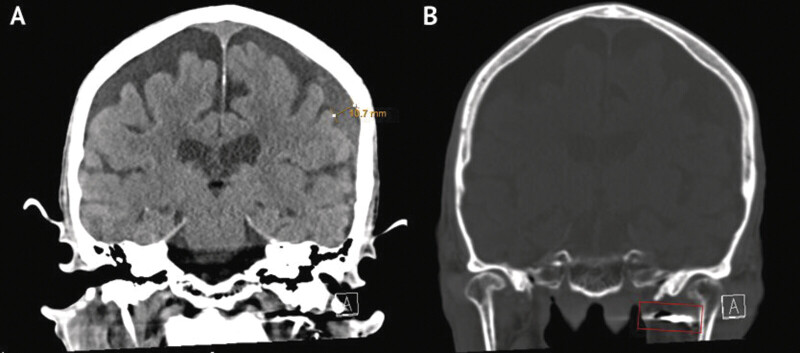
Postoperative head CT. (
